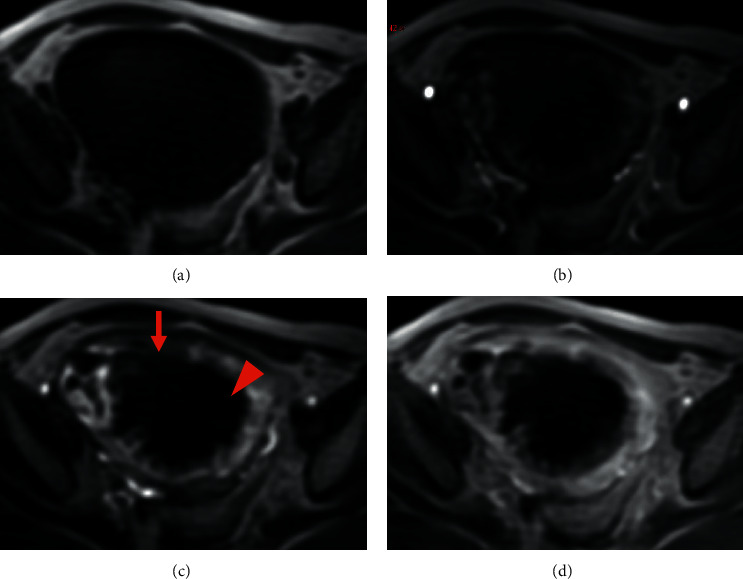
Pelvic MRI of leiomyosarcoma: (a, b, c, d) axial DCE-MRI without injection (a), at arterial phase (b), venous phase (c), and late phase (d). Note the disruption of the peripheral vascular ring (arrow) and heterogeneity due to central necrosis (arrowhead) (c).
